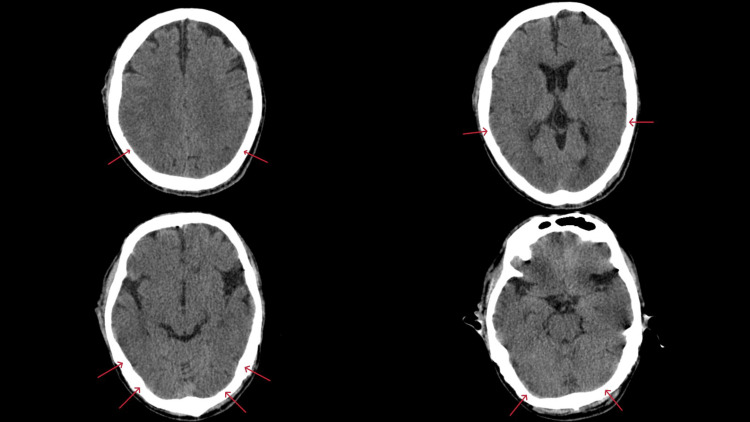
CT scan images on arrival show diffuse brain edema The sulci are markedly absent (red arrows), suggesting diffuse brain edema.
